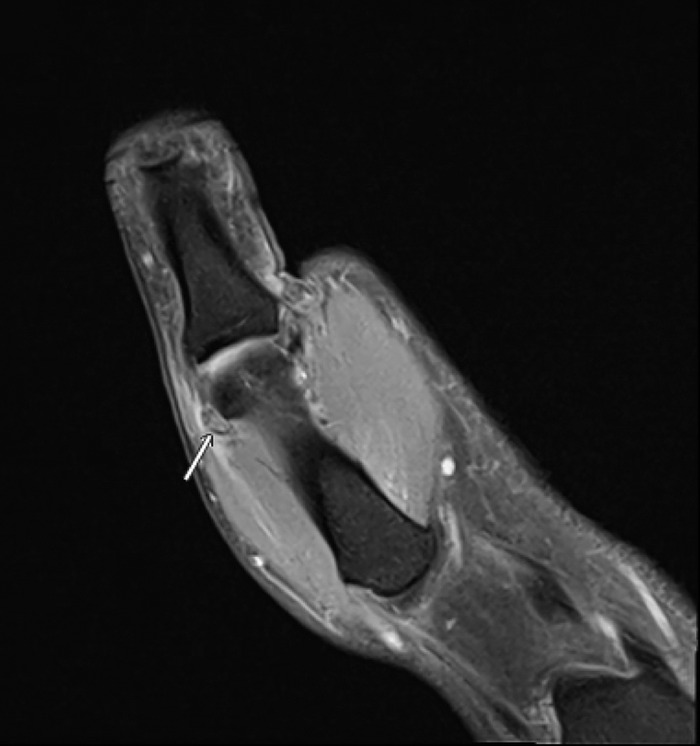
Preoperative magnetic resonance imaging of the right thumb shows the proximal radial collateral ligament tear with soft tissue interposition into the abductor pollicis brevis aponeurosis (arrow).
